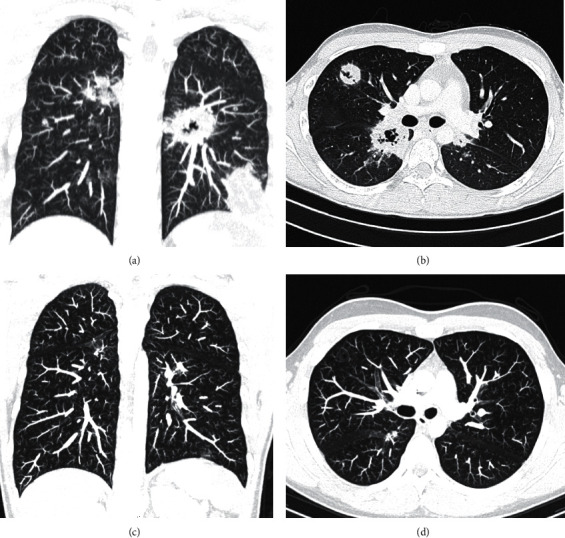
CT scan showing parenchymal nodular areas with internal cavitation (a, b) with almost complete resolution after 4 months (c, d), Case 1.
